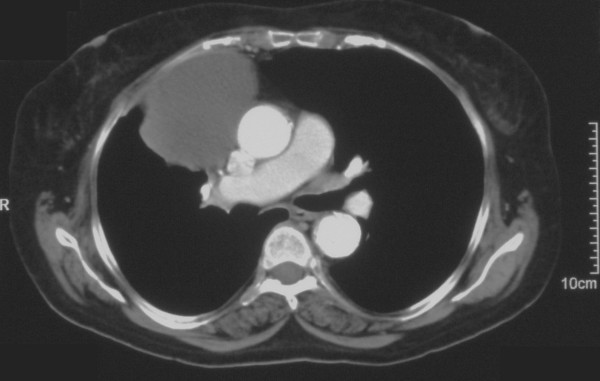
CT scan of chest showing a smooth ovoid low density mass abutting the right anterior chest wall, diaphragm and the right atrium and ventricle.
